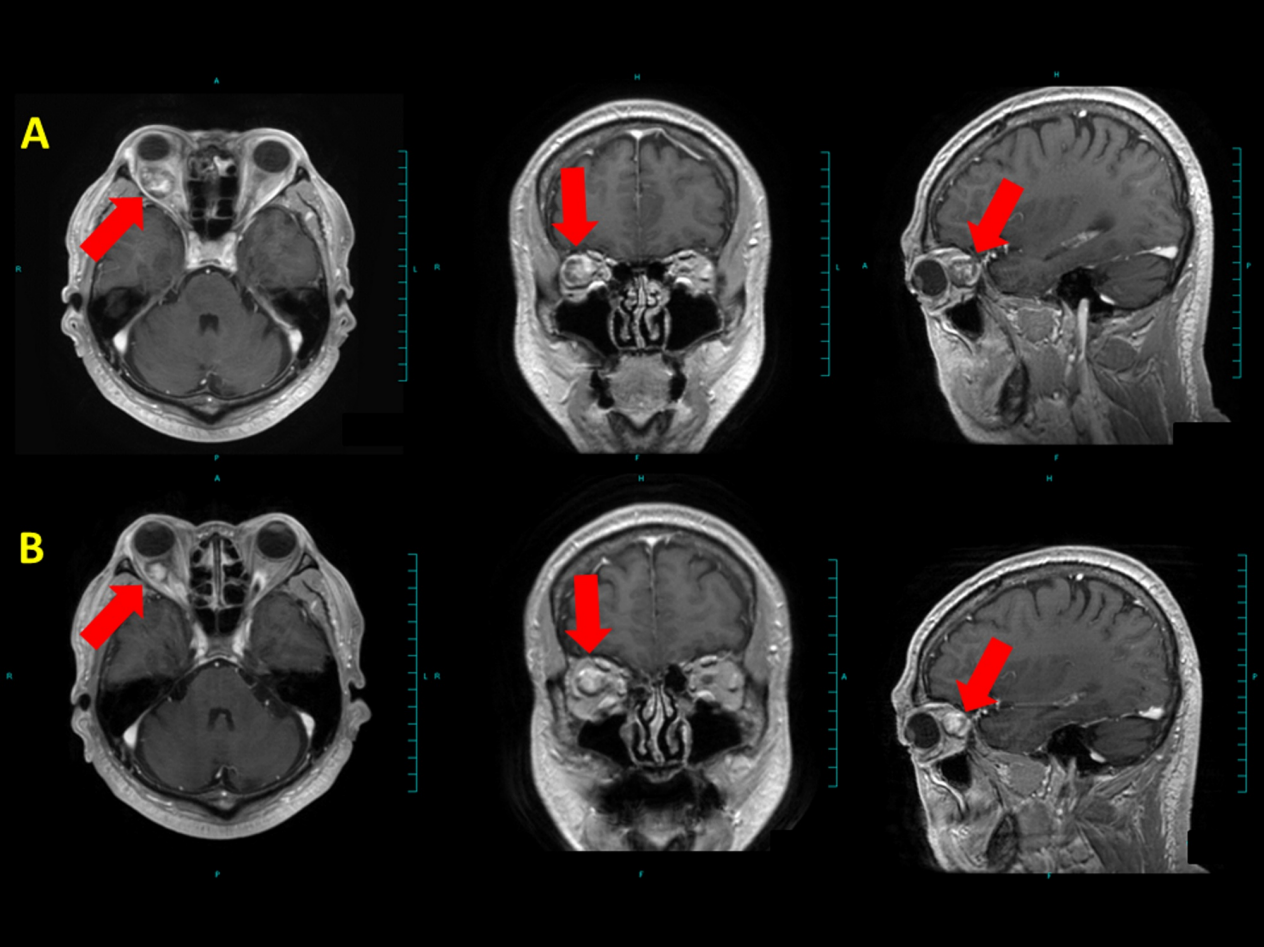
Brain MRI of case 3 Case 3 had decreased visual acuity and exophthalmos. (A) Before treatment and (B) 18 months after treatment. Red arrows denote tumor. The tumor decreased in size; visual acuity and exophthalmos were recovered.
